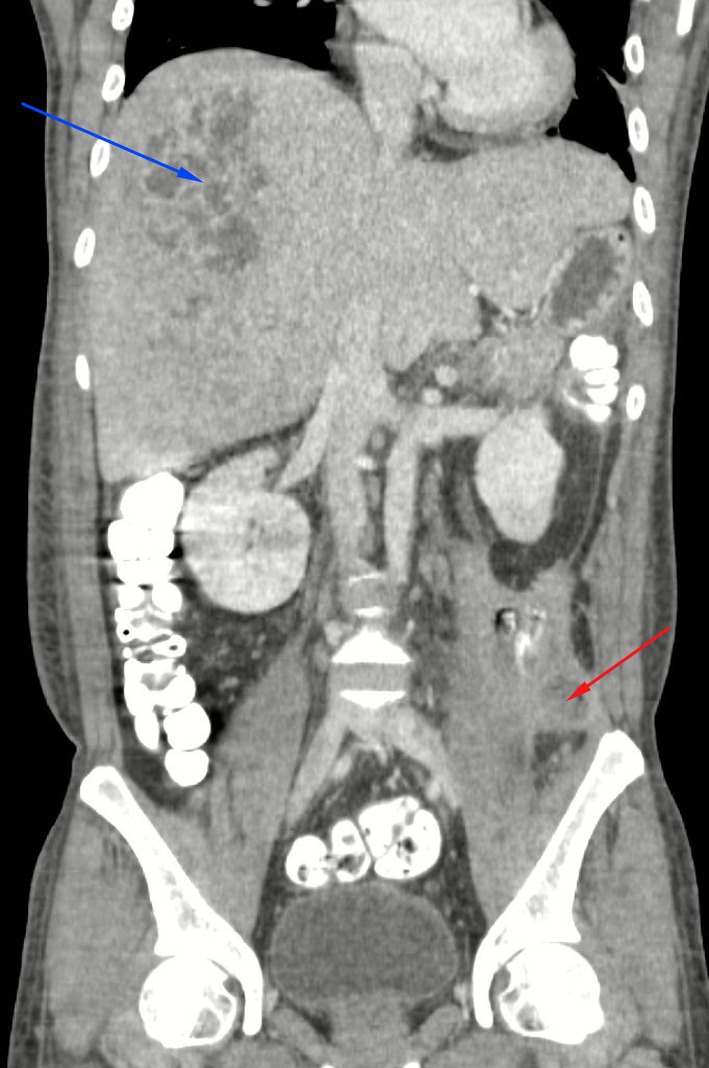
Computed tomography with oral and intravenous contrast of the abdomen and pelvis (coronal scan). Hypodense heterogeneous lesions corresponding to a large hepatic abscess (blue arrow) and pericolonic abscess (red arrow) were detected and subsequently treated with percutaneous drainage.
